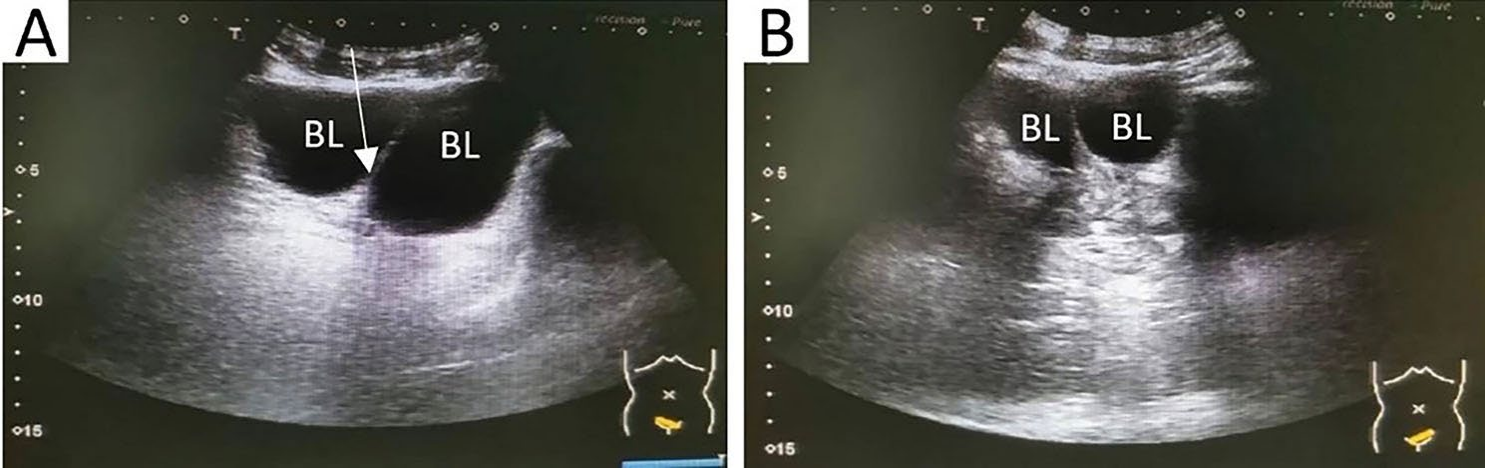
Urinary ultrasound: the left and right bladders (BL) were not connected, which were separated by a septum (white arrow)
